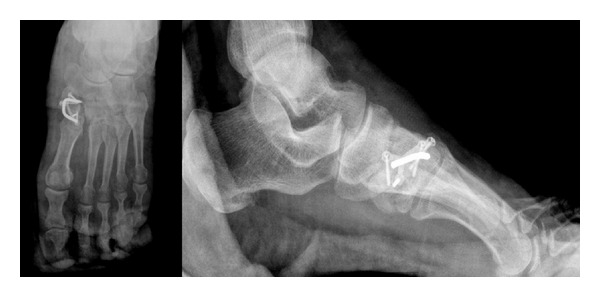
Postoperative anteroposterior and lateral direct X-ray.
